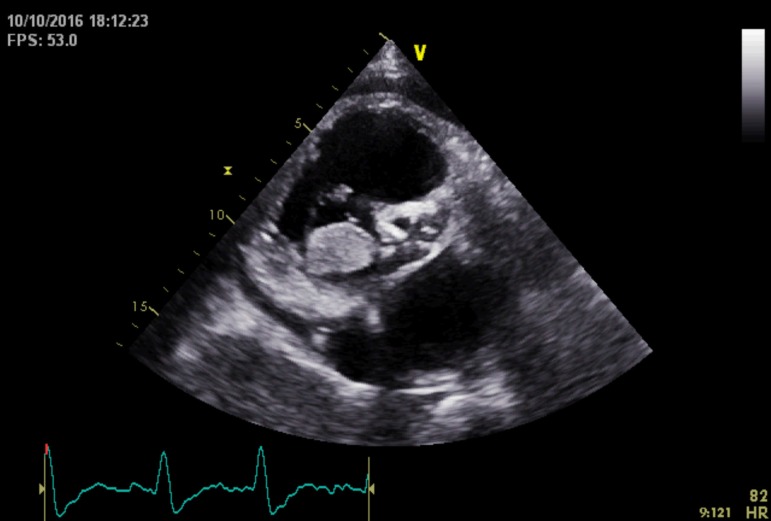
Echocardiogram revealed a large mass in the right ventricle prolapsing into
